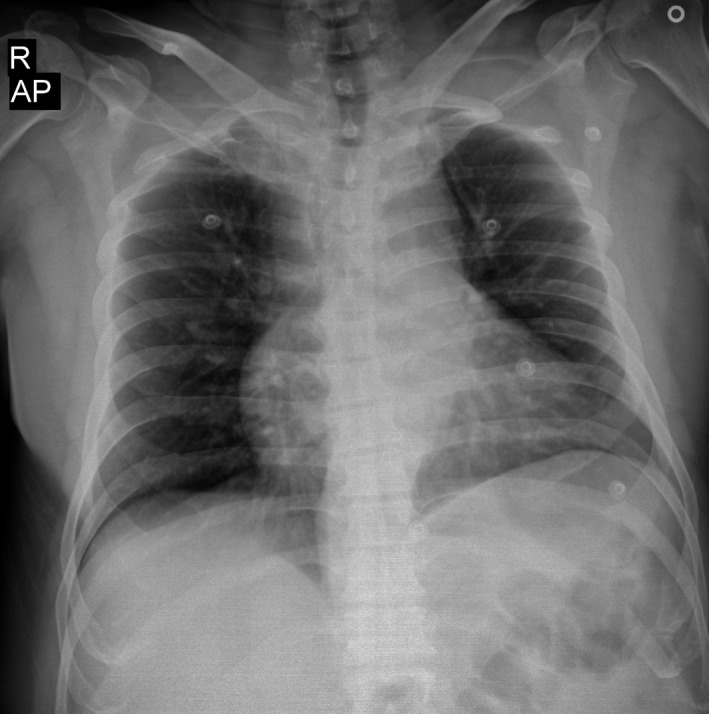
Chest radiograph demonstrating a widened mediastinum
